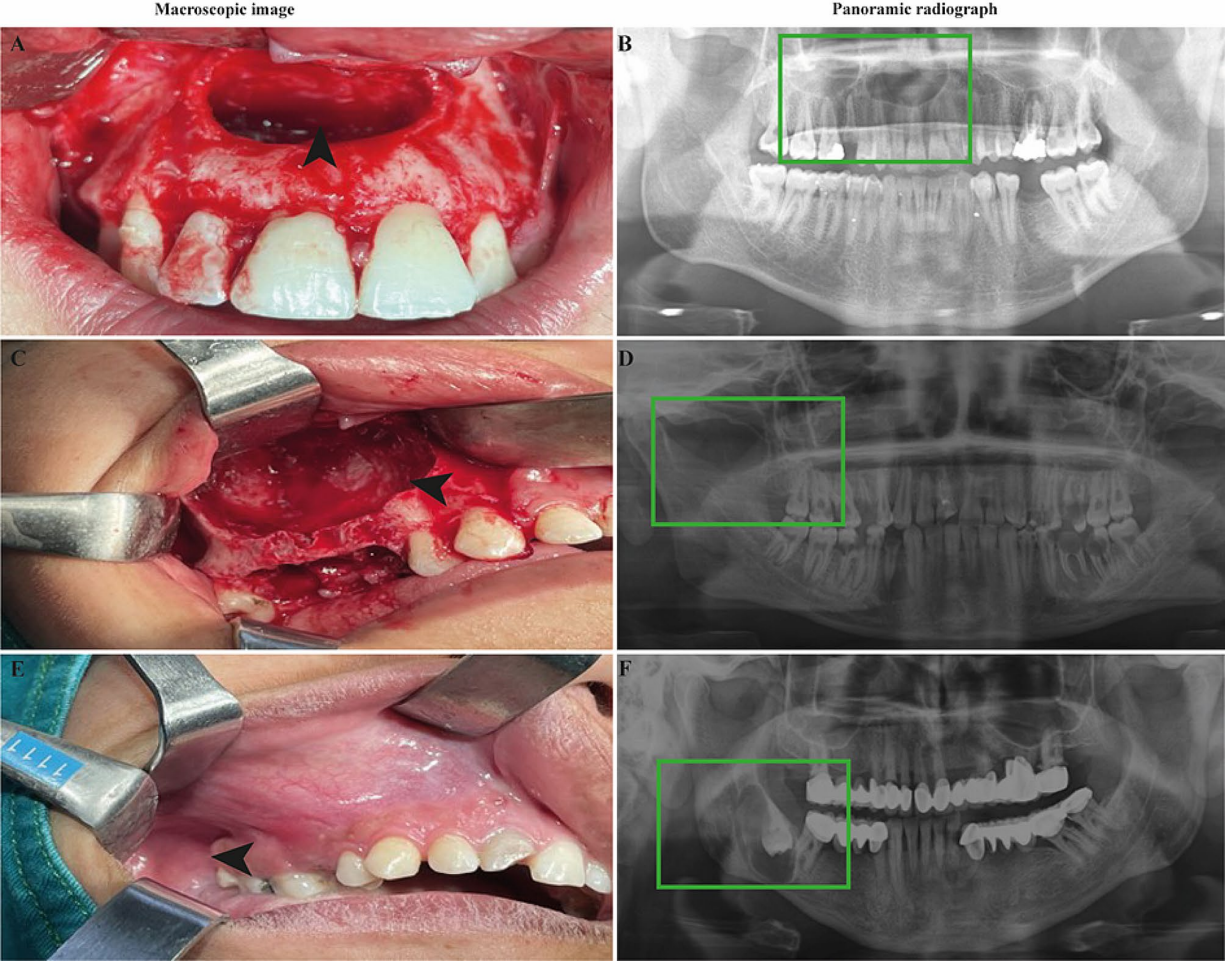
Macroscopic and panoramic radiographical images of patients with odontogenic cysts. The cystic area in macroscopic images (arrowhead) and in panoramic radiographies (green rectangle) in odontogenic keratocyst (A and B, respectively), radicular cyst (C and D, respectively) and dentigerous cyst (E and F, respectively)
